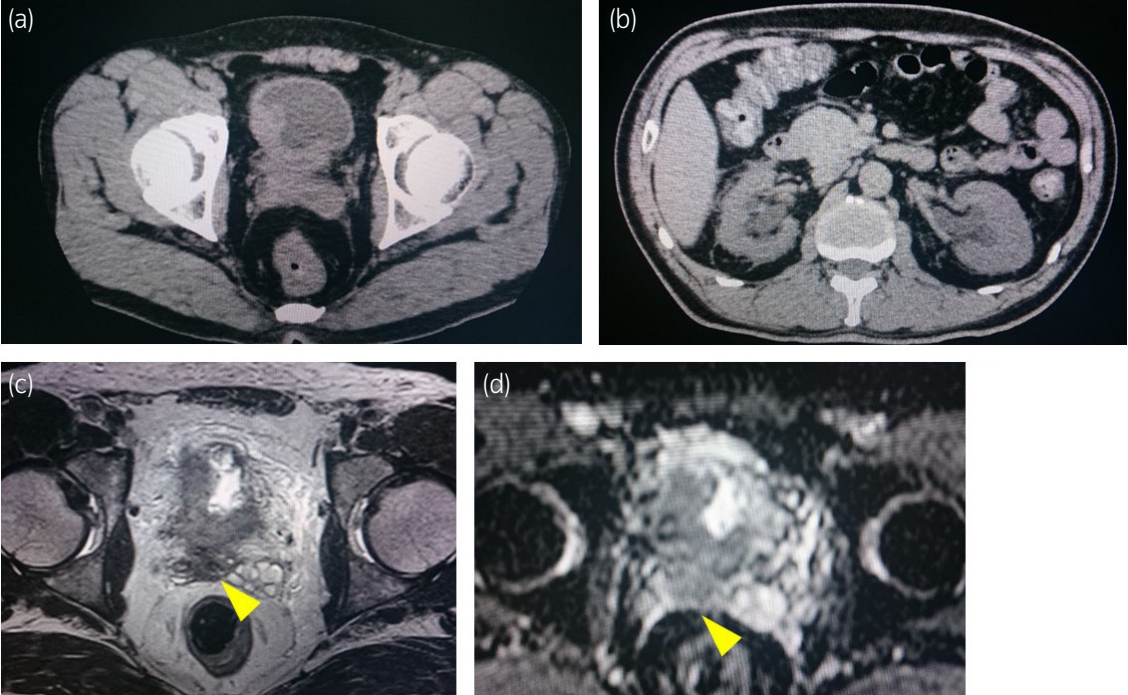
Results of preoperative abdominal CT (a, b) and pelvic contrast‐enhanced MRI (c, d). (a, b) Irregular thickening of the bladder wall (a) and bilateral hydronephrosis (b) are seen because bladder tumor involves bilateral ureteral orifices. (c, d) T2‐weighted imaging (c) and apparent diffusion coefficient mapping (d) show a large, irregular mass with significant extravesical invasion around the right wall and bladder trigone. Bladder tumor involves bilateral ureteral orifices and has invaded into the prostate and right seminal vesicle (yellow arrows). Clinical stage T4a bladder cancer was diagnosed.
